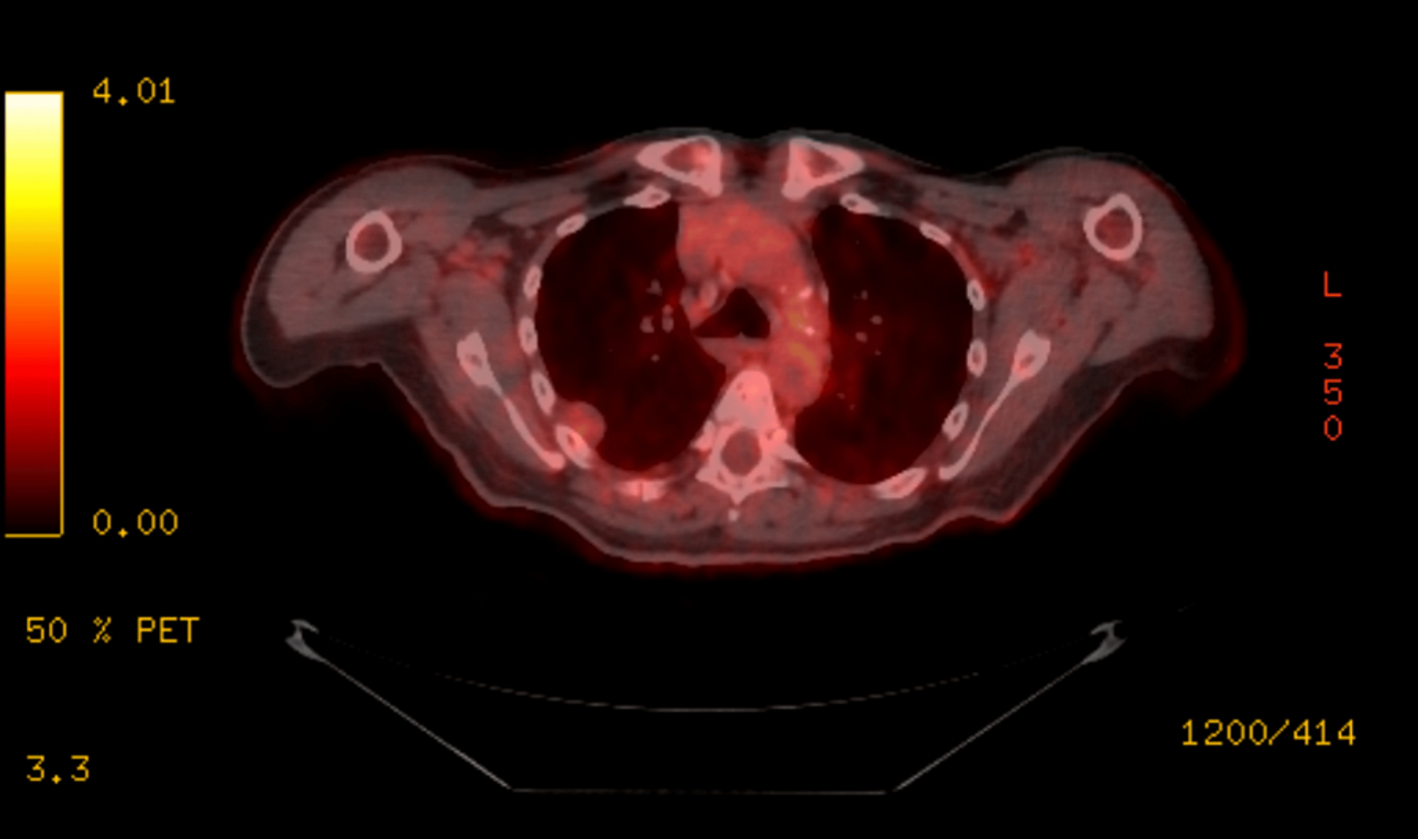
An 18-FDG PET/CT finding of a hypermetabolic, mildly active right upper pleural-based nodule, with the highest SUV of 3.8. FDG, fluorodeoxyglucose; SUV, standardized uptake value; PET/CT, positron emission tomography/computed tomography
